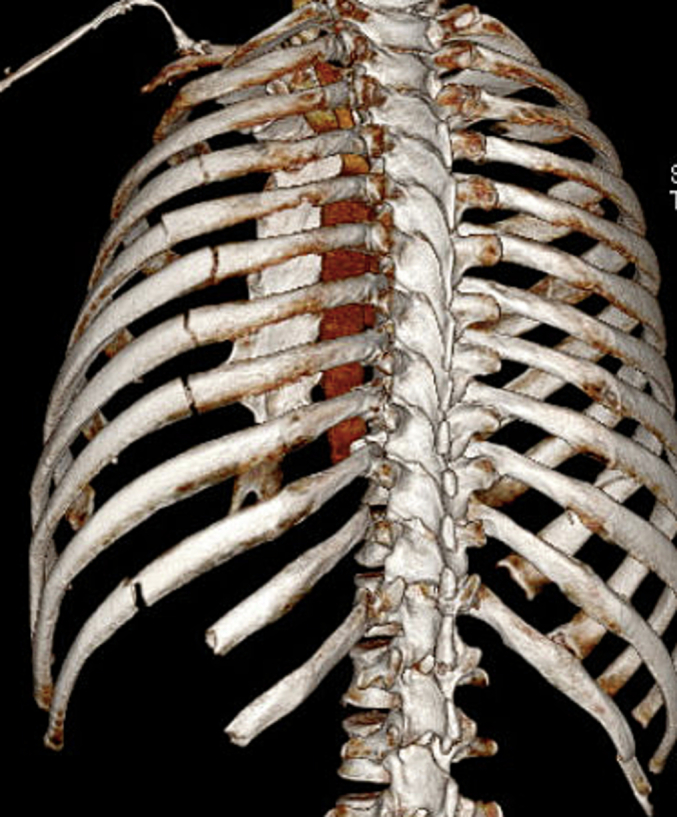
Preoperative CT chest with 3D Reconstruction demonstrating left-sided rib fractures.
